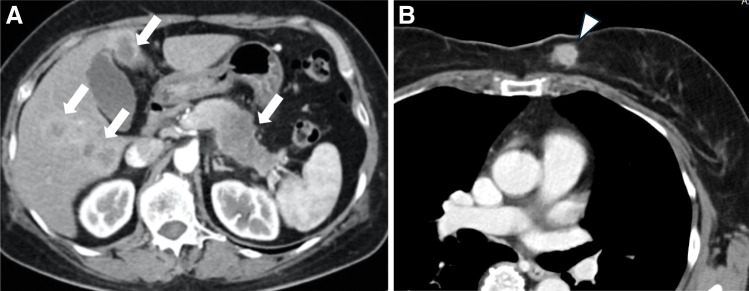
CT images. (A) Abdominal contrast-enhanced CT revealed a low-density mass occupying the entire pancreatic tail (white arrow) and multiple low-density masses in the liver (white arrows). (B) A 1.5-cm contrast-enhancing mass was identified in the lower inner quadrant of the left breast (white arrowhead).
